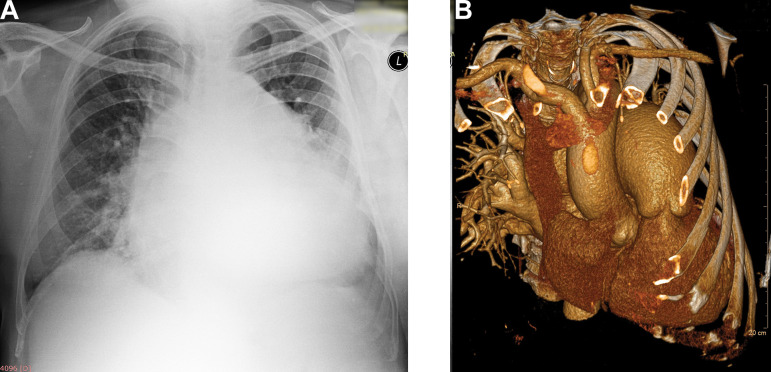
A) preoperative radiography; B) preoperative MSCT.
